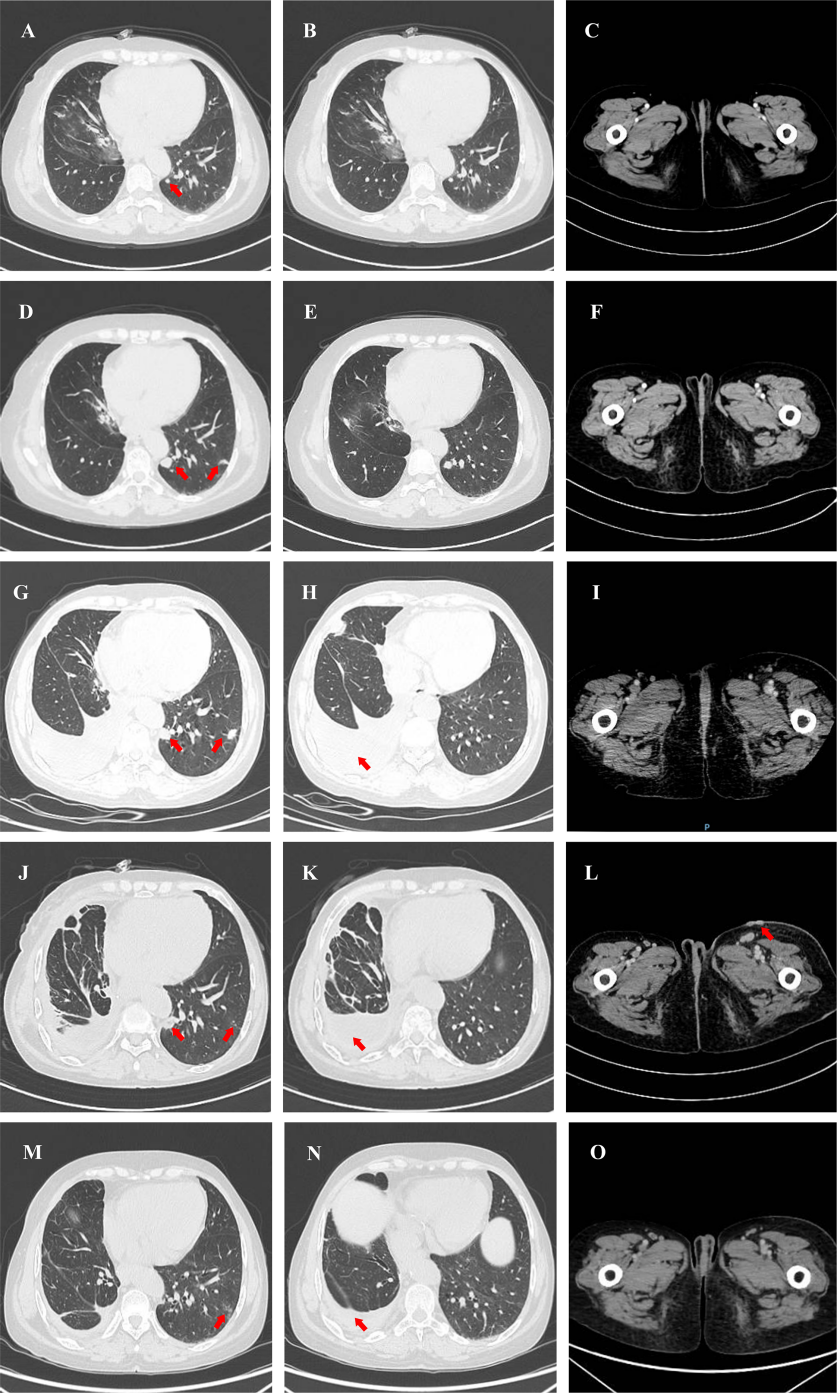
CT images of pulmonary tumor, pleural effusion, and inguinal region during treatment. (A)New pulmonary tumor after 23 months of icotinib. (B) No pleural effusion after 23 months of icotinib. (C) No abnormalities in the inguinal region after 23 months of icotinib. (D) Pulmonary tumor after 33 months of icotinib. (E) Pleural effusion after 33 months of icotinib. (F) No abnormalities in the inguinal region after 33 months of icotinib. (G) Pulmonary tumor after 31 months of befotertinib. (H) Pleural effusion after 31 months of befotertinib. (I) Inguinal region after 31 months of befotertinib. (J) Pulmonary tumor after 2 months of anlotinib. (K) Pleural effusion after 2 months of anlotinib. (L) Inguinal region after 2 months of anlotinib. (M) Pulmonary tumor after 21 months of crizotinib. (N) Pleural effusion after 21 months of crizotinib. (O) No abnormalities in the inguinal region after 21 months of crizotinib.
